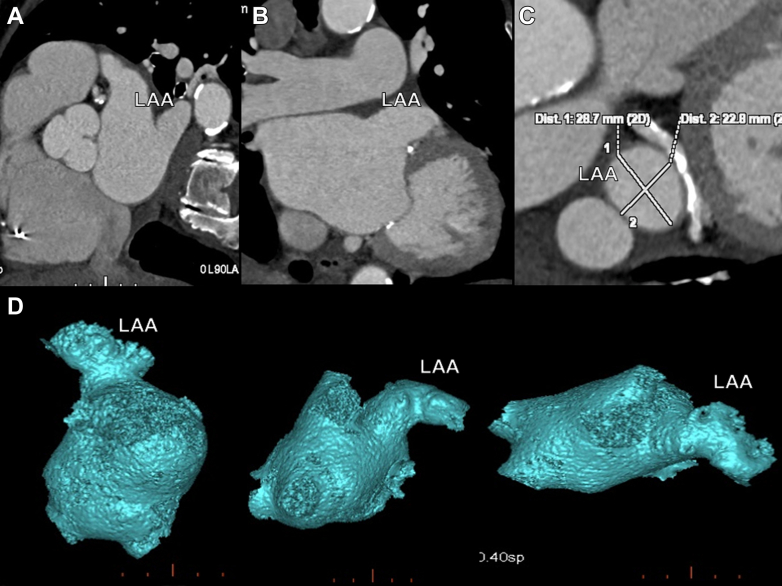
Preprocedural Computed Tomography Angiography (A to C) Multiplanar CTA views showing LAA morphology and excluding thrombus. (D) Three-dimensional reconstruction of LAA. CTA = computed tomography angiography; LAA = left atrial appendage.
